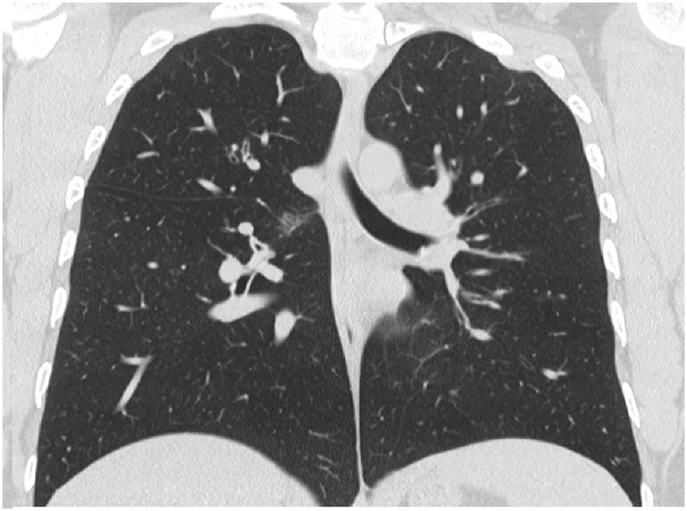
September 2020 CT chest lung window near normal.
